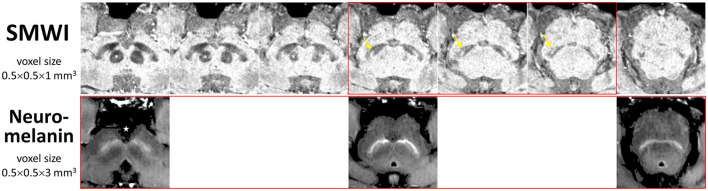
Anatomical landmarks for slice selection for qualitative visual evaluation and segmentation of the substantia nigra for quantitative image analysis. Corresponding consecutive (cranio-caudal—left-to-right) midbrain iron- (SMWI) and neuromelanin-sensitive MRI slices demonstrate the relations of the three slices (boxed in red) used between modalities. Loss of the normal hyperintense nigrosome-1 within the hypointense substantia nigra (yellow arrow) was assessed on SMWI slices just below the inferior pole of the red nucleus (asterisk). Loss of normal hyperintensity in the substantia nigra was assessed on neuromelanin-sensitive slices from the last slice through the interpeduncular fossa (star). Permission had been granted by the participating control subject for the publishing of these images.
